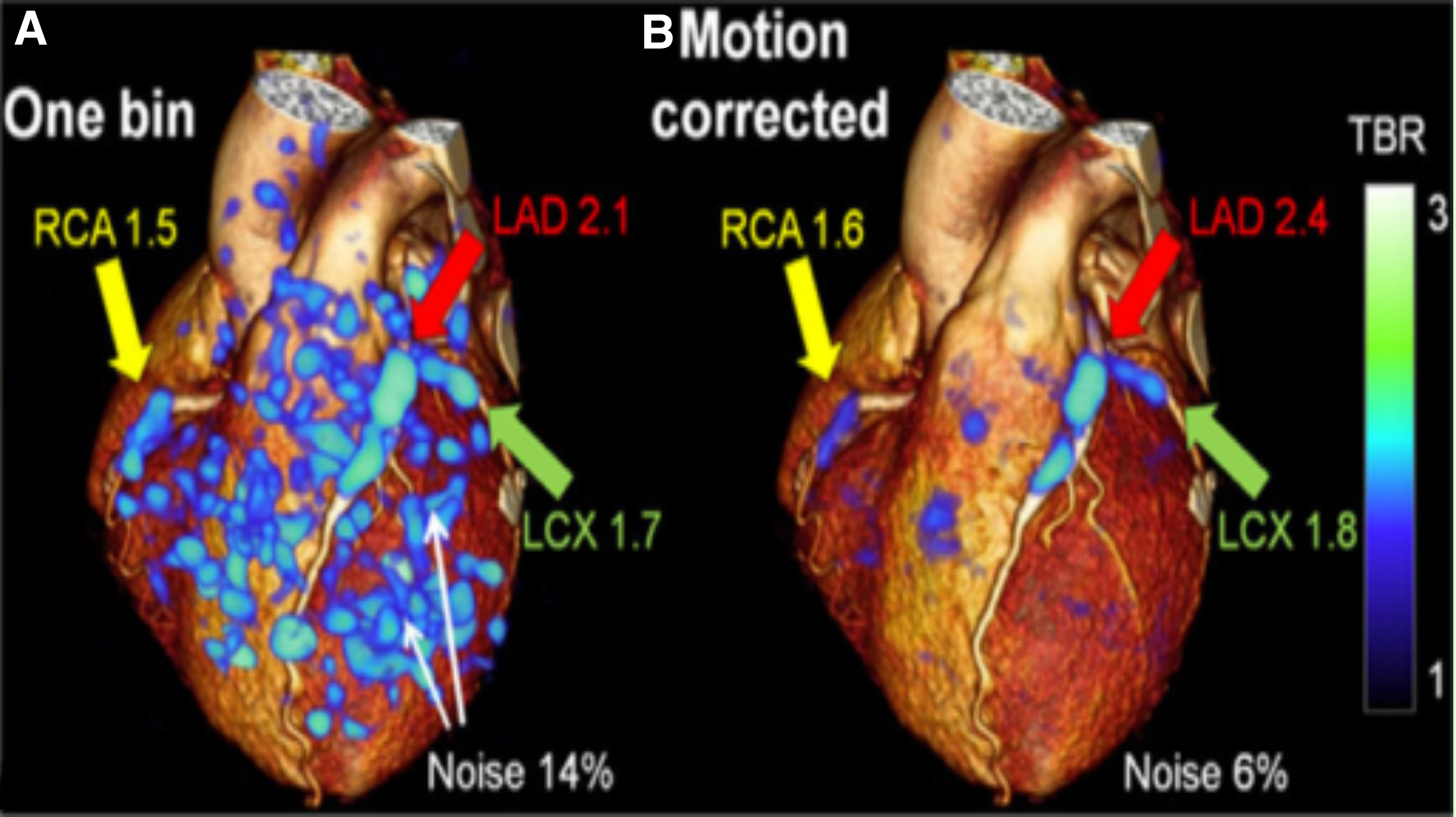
3D CT-PET: Target-to-background refinement and noise reduction with (A) The 1-bin image (25% of PET counts) (90); (B) Motion-corrected image overlayed on rendered CCTA volume. Increased plaque update is seen in RCA, LAD, and LCX coronary arteries in the high noise 1-bin image and remains clear in the motion-corrected image. RCA, right coronary artery, LAD, left anterior descending, LCX, left circumflex artery (91).
